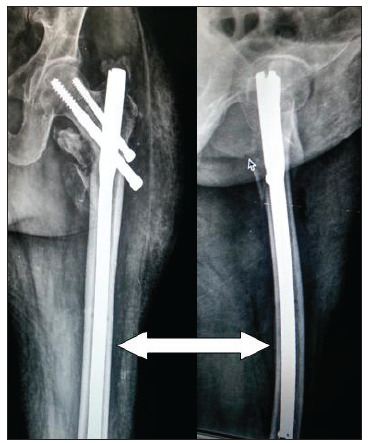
Postoperative radiographs produced after revision
